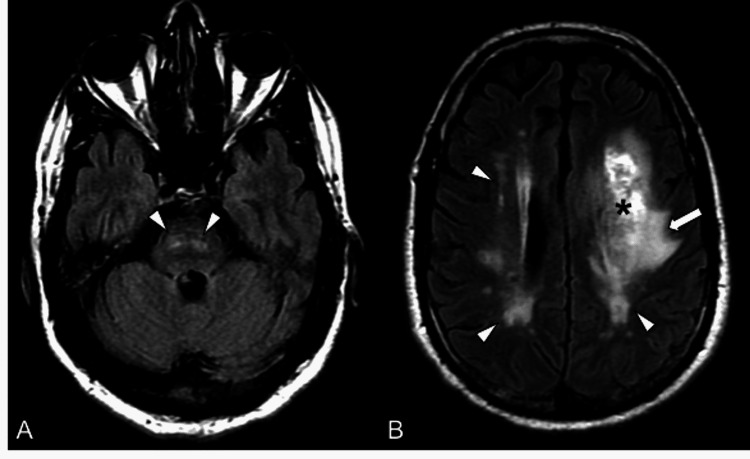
Magnetic Resonance Imaging Workup (A) Axial fluid-attenuation-recovery (FLAIR)-weighted images at the pontine level reveal patchy hyperintense signal intensities within the pons (arrowheads). (B) Axial FLAIR-weighted images at the level of the cerebral hemispheres show bilateral periventricular, patchy deep white matter hyperintensities (arrowheads) as well as edematous changes (white arrow) associated with the left basal ganglia hematoma (black asterisk). The patchy hyperintense signal abnormalities in the cerebral hemispheres and pons are most consistent with small vessel ischemic changes.
